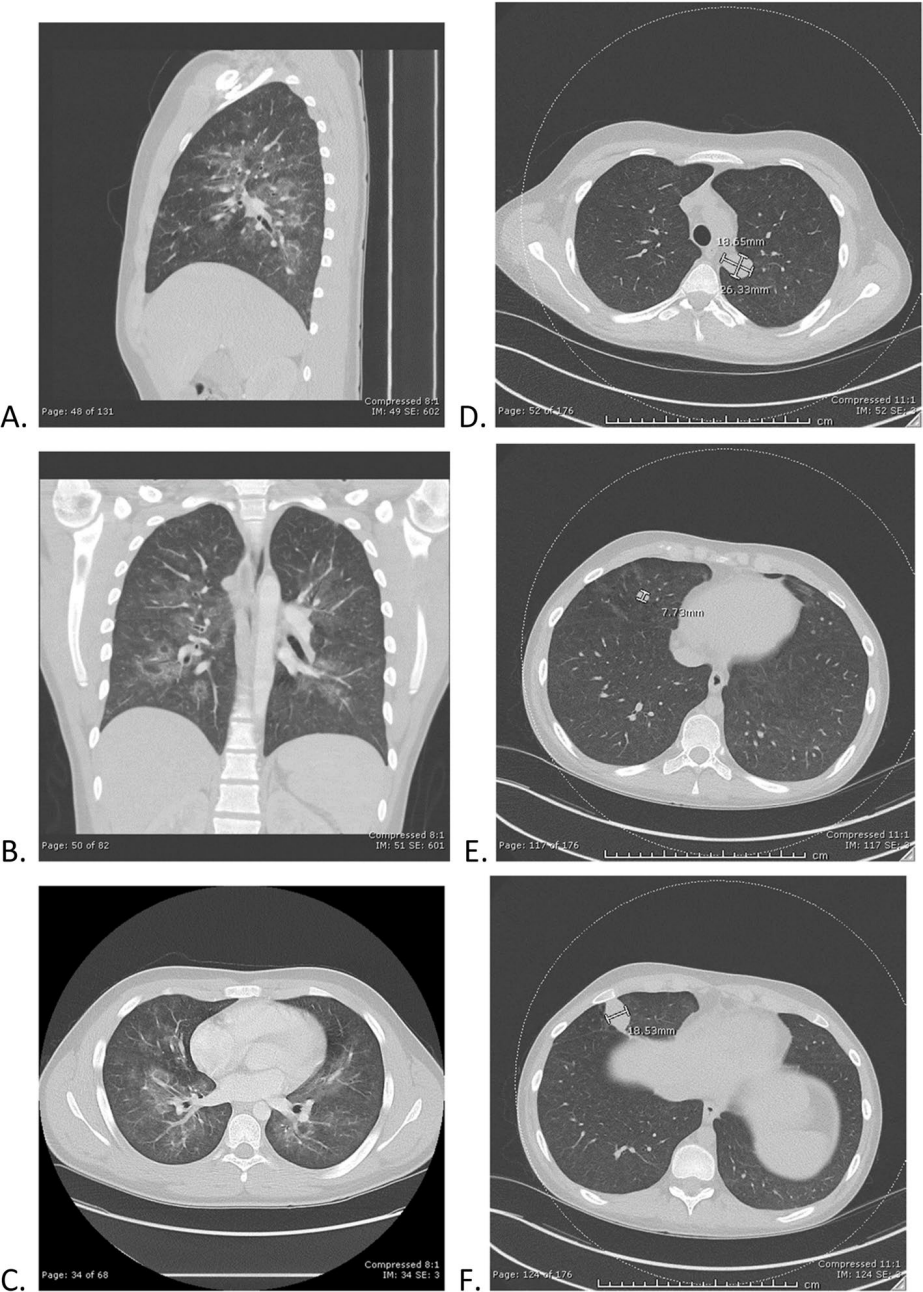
CT scan findings for patient 1 (A-C) and patient 2 (D-F). A, B, C: CT chest of Patient 1. Final read: Ill-defined, confluent groundglass attenuation is present bilaterally in the lungs, roughly following the bronchovascular bundles. No dense consolidation is seen. No pleural effusions are identified. D, E, F: CT Chest of Patient 2. Final read: Subtle diffuse symmetric ground glass opacities throughout both lungs. Findings may represent pulmonary hemosiderosis related to sequela of prior diffuse bilateral pulmonary hemorrhage. Two well defined solid appearing soft tissue density nodules within the right middle lobe with surrounding tiny parenchymal cysts are present. Another similar appearing well defined nodule centered in the upper aspect of the major fissure of the left lung where it contacts the mediastinum is present. These nodular opacities are without cavitation or calcification and were not clearly seen on prior portable chest radiographs. Evaluation is limited due to the lack of IV contrast and their etiology is unclear. The tiny parenchymal cysts adjacent to the nodules in the right middle lobe could represent sequela of a necrotizing lung process. These nodules could represent organized parenchymal or pleural hematomas. The appearance is not highly suggestive of infection due to lack of surrounding inflammation. Neoplasm seems unlikely given the history
